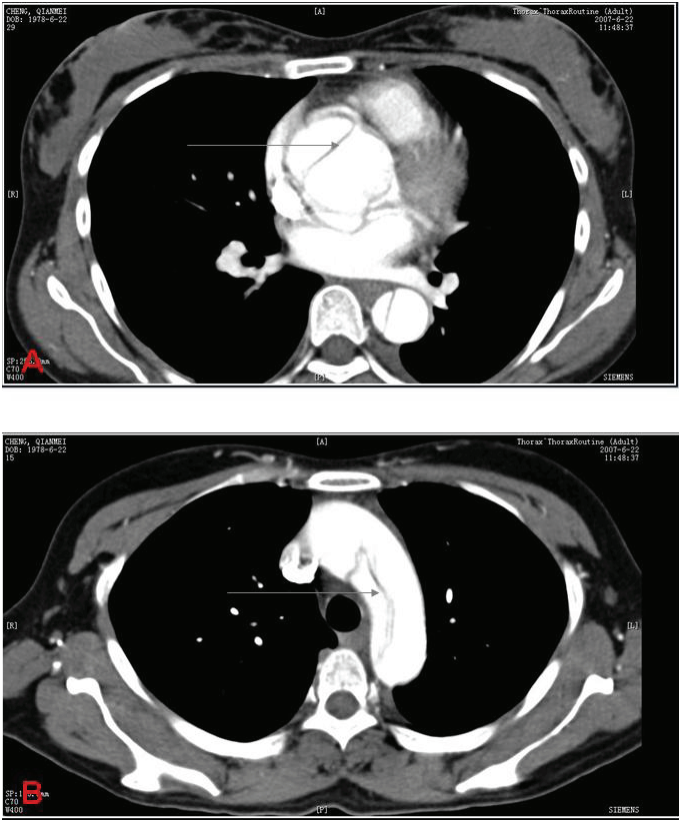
Aortic dissection in case I with Marfan syndrome. (A) CT scan: Ascending aortic dissection (grey arrow), (B) CT scan: Aortic arch dissection (grey arrow).
